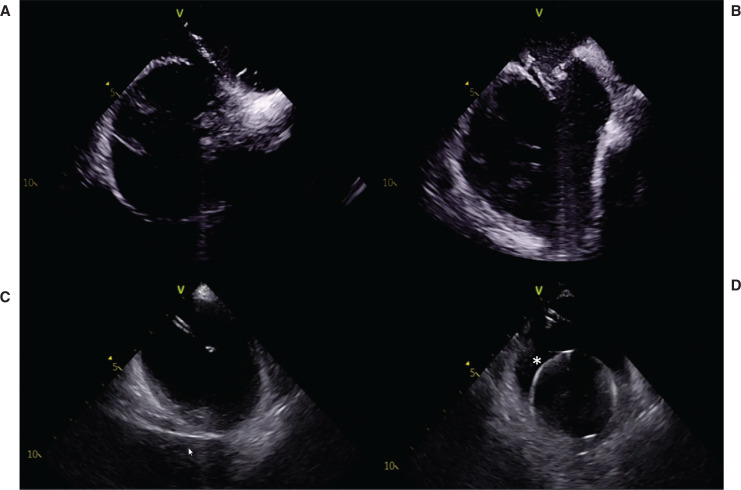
Intracardiac echocardiography showing the steps for a successful transseptal puncture. The transseptal system (sheath, dilator, and needle) is gradually withdrawn while maintaining the needle in the 4 o’clock position. A drop is typically felt after (A) the superior limbus of the interatrial septum has been reached, and another one is noted at the level of the fossa ovalis. B: The needle is then advanced to ensure “tenting” as it is exposed. Once the transseptal puncture has been performed, left atrial pressure is obtained to ensure safe placement. The needle is then held in place while (C) the dilator is advanced over it before (D) being exchanged for a ProTrack pigtail wire, as indicated by the asterisk. The arrow in (C) points to the aorta.
